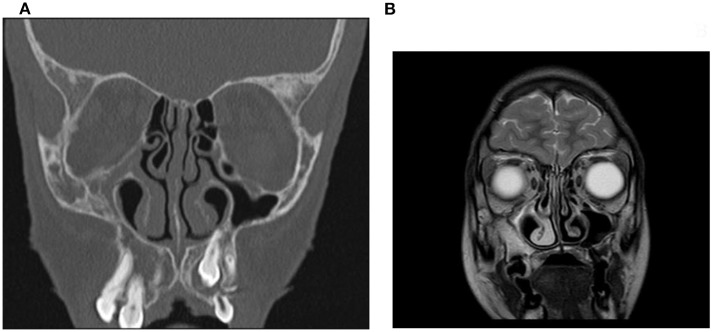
Facial MRI (A) and CT (B) scans performed in the patient with WS when she was 7 years old. MRI showed that the right maxillary sinus was opacified, with central inspissated secretions; it was also diminished in volume with inward bowing of the maxillary walls and the uncinate process was lateralized (A). CT scan confirmed the opacified right maxillary sinus with retraction of the maxillary walls as well as the lateralized and demineralized uncinate; it also demonstrated the partial occlusion of the maxillary infundibulum and the fact that the orbital floor was located inferiorly.
